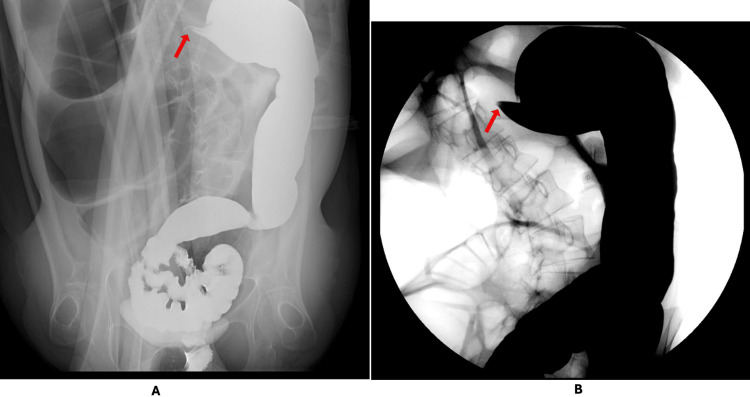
Water-soluble contrast enema on hospital day one (A) Abdominal radiograph showing contrast opacification of the colon with abrupt narrowing at the distal transverse colon (arrow). (B) Fluoroscopic image demonstrating an abrupt bird’s beak taper at the distal transverse colon (arrow), consistent with mechanical torsion.
